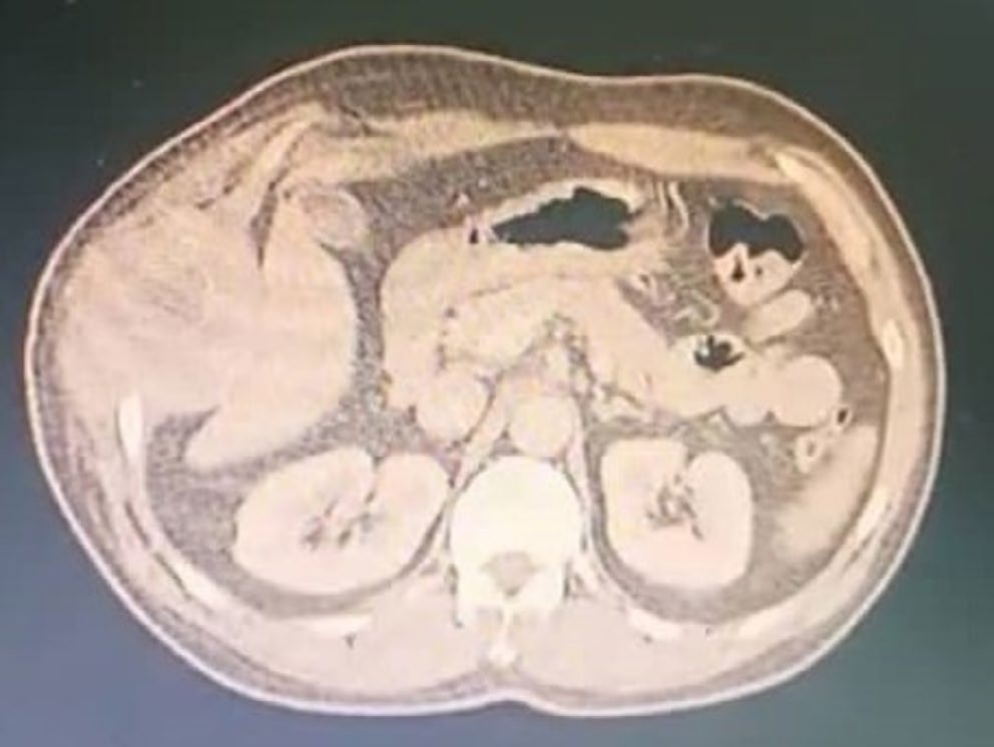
Axial CT at a slightly different level. The chest wall mass remains in broad contact with the right hemidiaphragm, with effacement of the intervening fat plane—features concerning diaphragmatic involvement. No pleural effusion is evident on these cuts.
